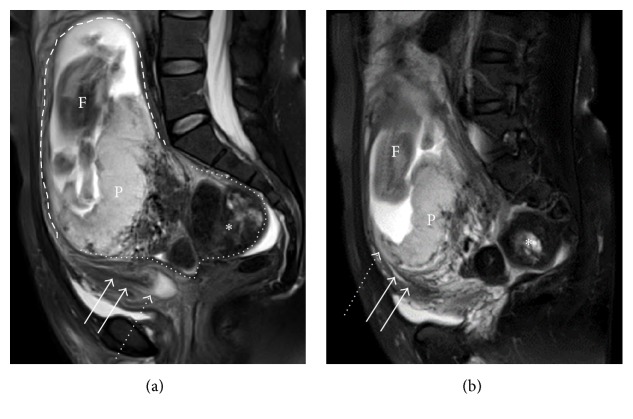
Sagittal T2-weighted magnetic resonance imaging (MRI) at 18 weeks of gestation shows a large fibroid (white asterisk) engaged in the pouch of Douglas. The cervix (white arrow) and the anterior wall of uterus (dashed line) are cranioventrally stretched. The fundus and the posterior wall of uterus (dotted line) were entrapped in the pelvis between the sacral promontory and pubic symphysis. Dashed arrows show external ostium of uterus (a) and internal one (b). F = fetus; P = placenta (a, b).
